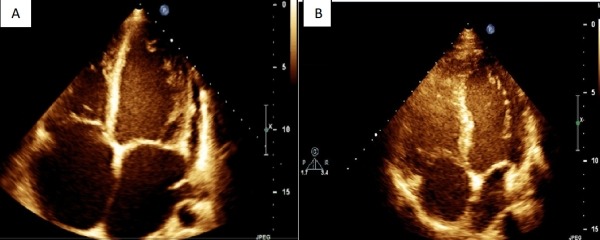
Echocardiographic image apical four-chamber of left ventricle in end-diastole before (A) and after (B) of ablation showing decreasing volumes.
